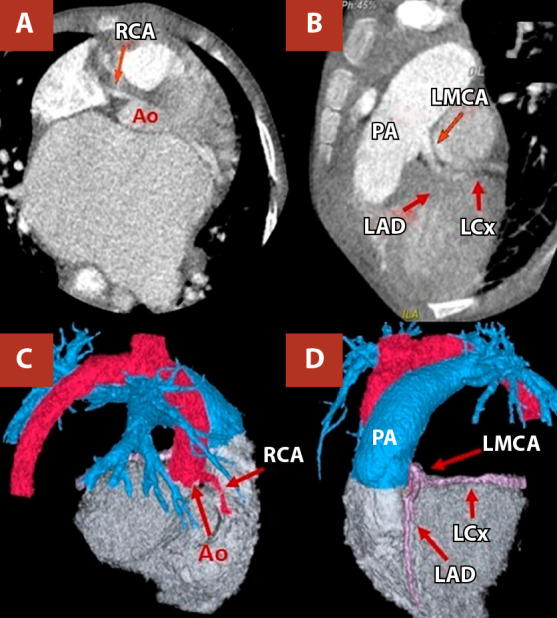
Coronary computed tomography angiography. (A) Normal origin of the right coronary artery from the ascending aorta. (B) The left main coronary artery arises anomalously from the pulmonary artery. (C, D) Three-dimensional reconstruction shows the normal course of the right coronary artery and the anomalous origin of the left main coronary artery
